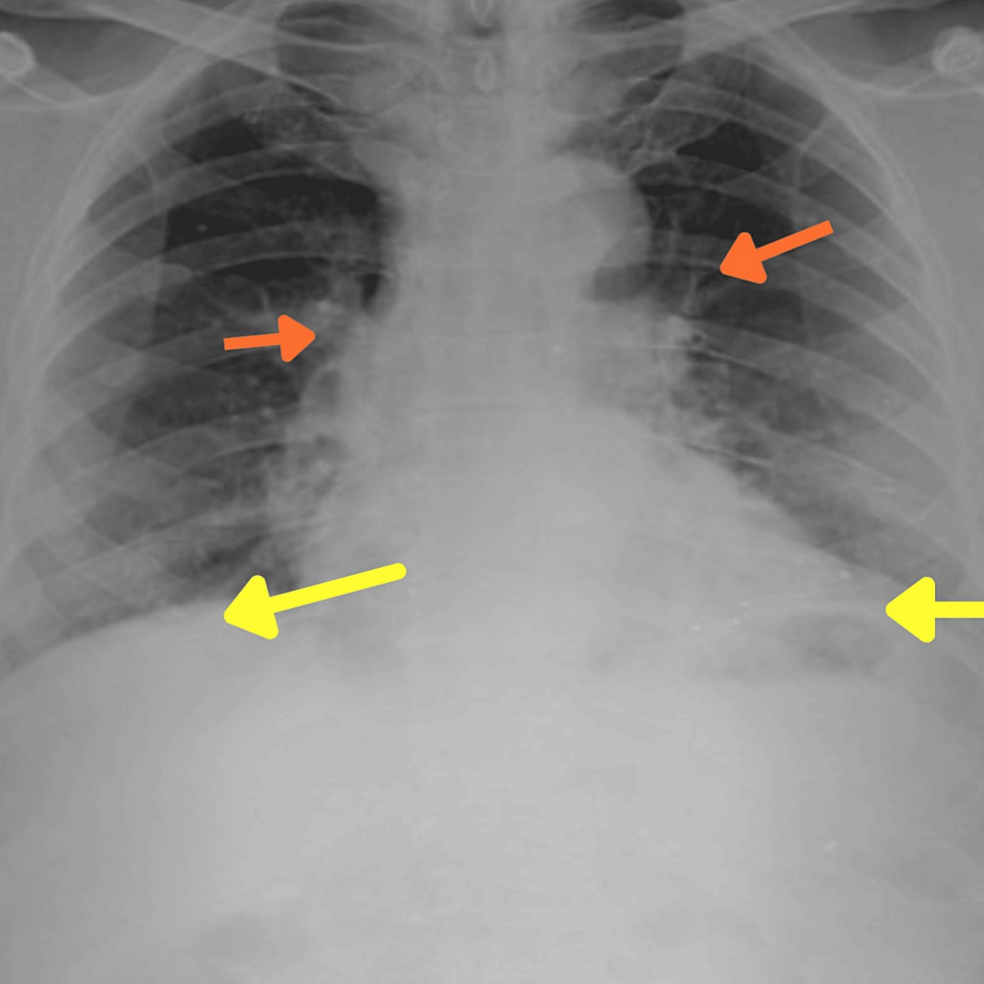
Chest radiograph Flattened diaphragm (yellow arrows) and increased broncho-vascular markings (orange arrows).
